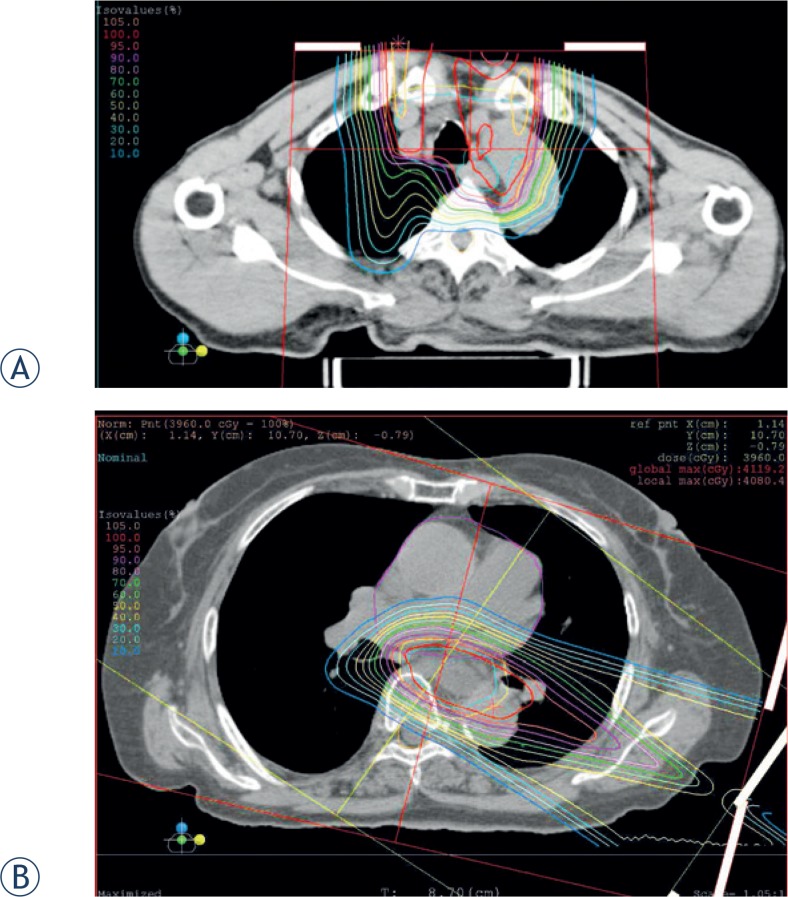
Dose distribution map for proton beam therapy following initial X-ray irradiation. The region outside the outermost line received <10% radiation. (A) Dose distribution map for cephalic esophageal cancer. (B) Dose distribution map for thoracic esophageal cancer.
